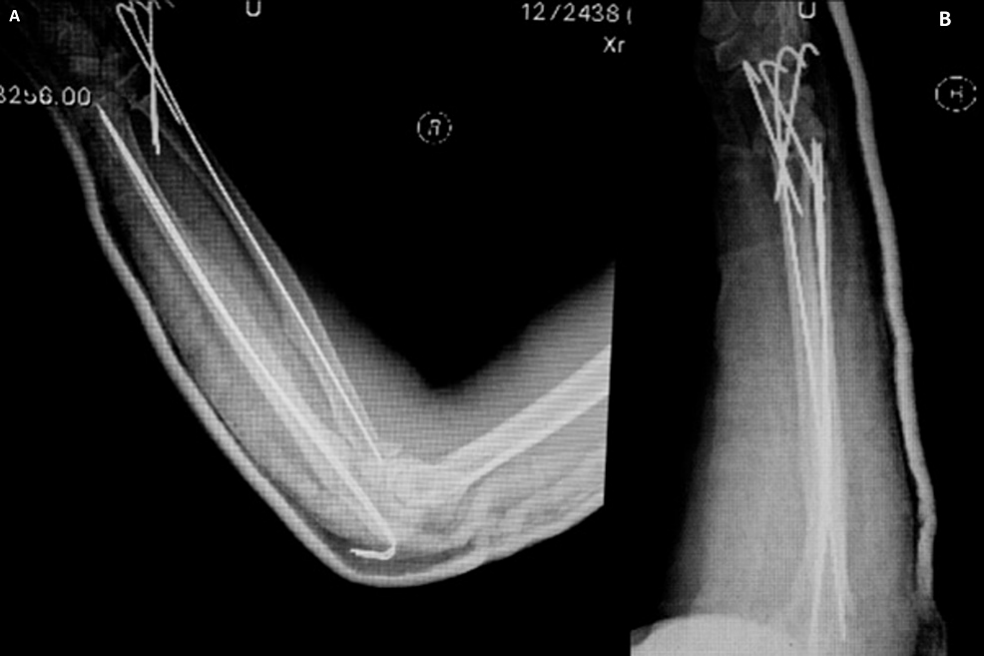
Postoperative anteroposterior (A) and lateral (B) radiographs of the forearm showed a good reduction and restored alignment. Three K-wires were used for the distal radius, and one intramedullary K-wire was used to fix the radial neck fracture. Two intramedullary wires were used to fix the olecranon and distal ulna fractures.
